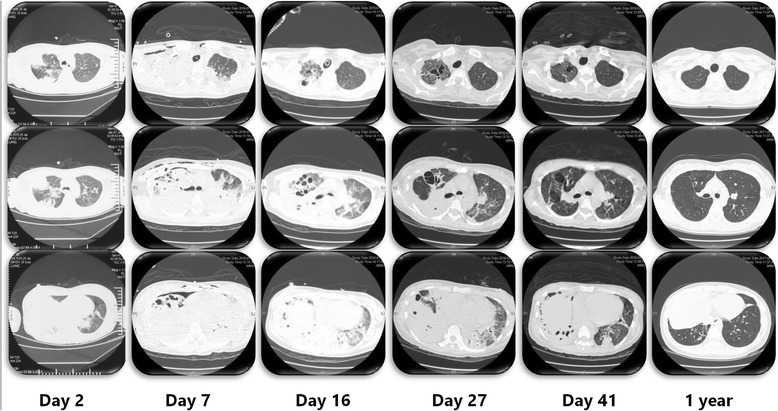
Chest computed tomography (CT) on day 2 before RICU admission and on day 7, day 16, day 27 and day 41 and followed up after 1 year. Three representative slices of the upper, middle and lower lobe were chosen
